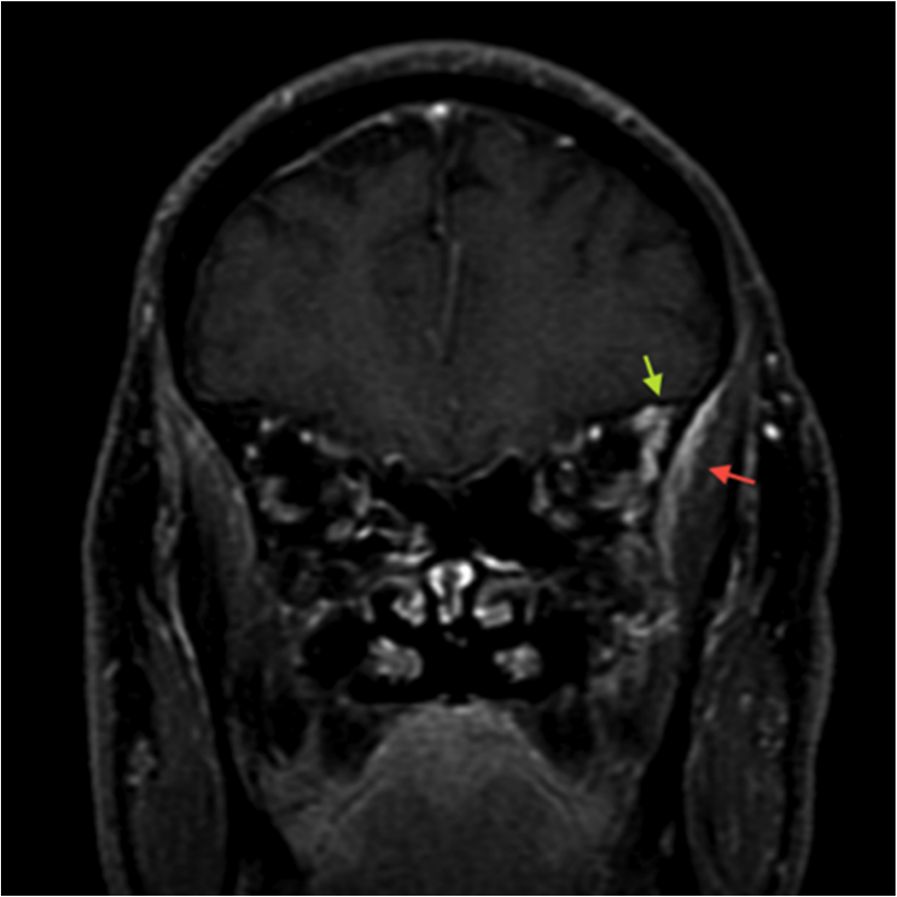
Coronal contrast-enhanced, T1-weighted magnetic resonance imaging scan. Contrast agent was injected with fat suppression mode on,, and enhancement of the left temporal muscle (red arrow) associated with ectasia of contiguous vessels (green arrow) is evident as an index of inflammation.
